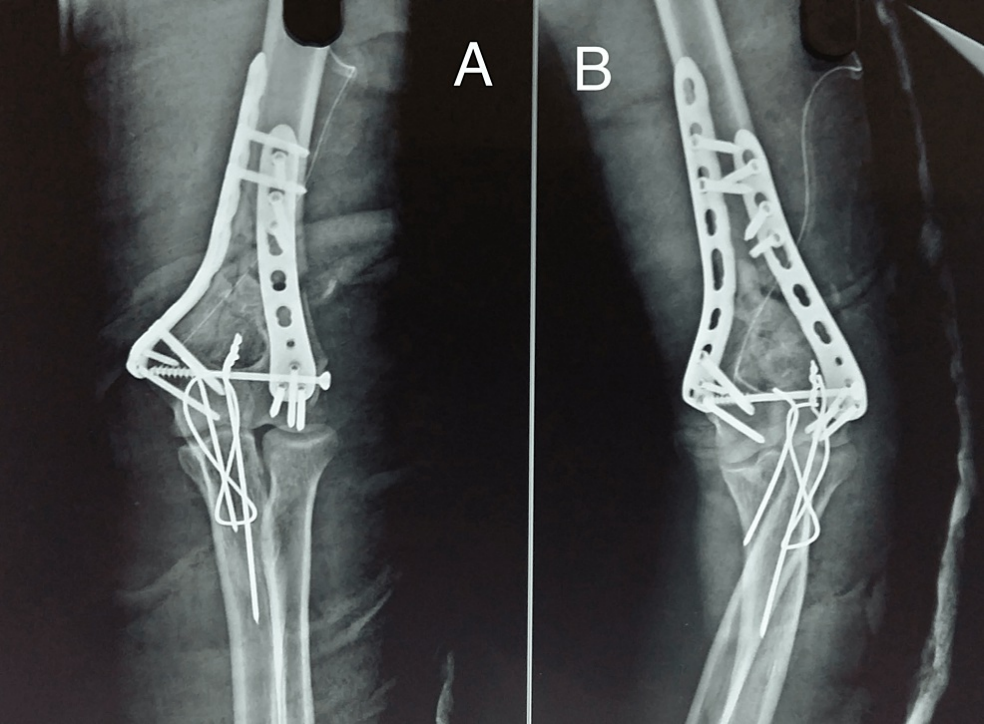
Postoperative radiograph. Immediate postoperative radiograph showing fixation of the fracture with distal humerus anatomical locking plates using the posterior olecranon osteotomy approach. A: anteroposterior view; B: lateral view.
